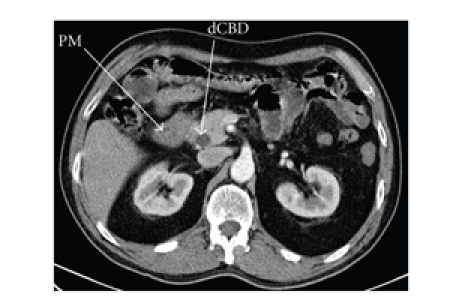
CT with contrast (arterial phase) showing the mass
